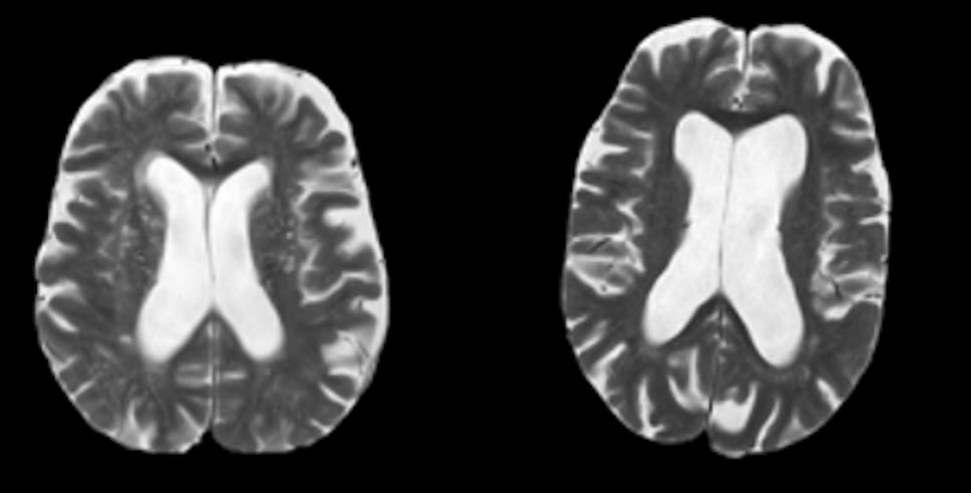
MRI visual assessment for enlarged perivascular spaces (ePVS) in two patients suffering from chronic adult hydrocephalus. ePVSs are seen on axial T2-weighted MRI, obtained at the level of the periventricular parenchyma, 20 mm above the anterior commissure, as linear-, ovoid- or round-shaped hyperintensities. The image on the left shows more than 11 ePVS (high ePVS burden), while the image on the right shows 10 or less ePVS (low ePVS burden).
